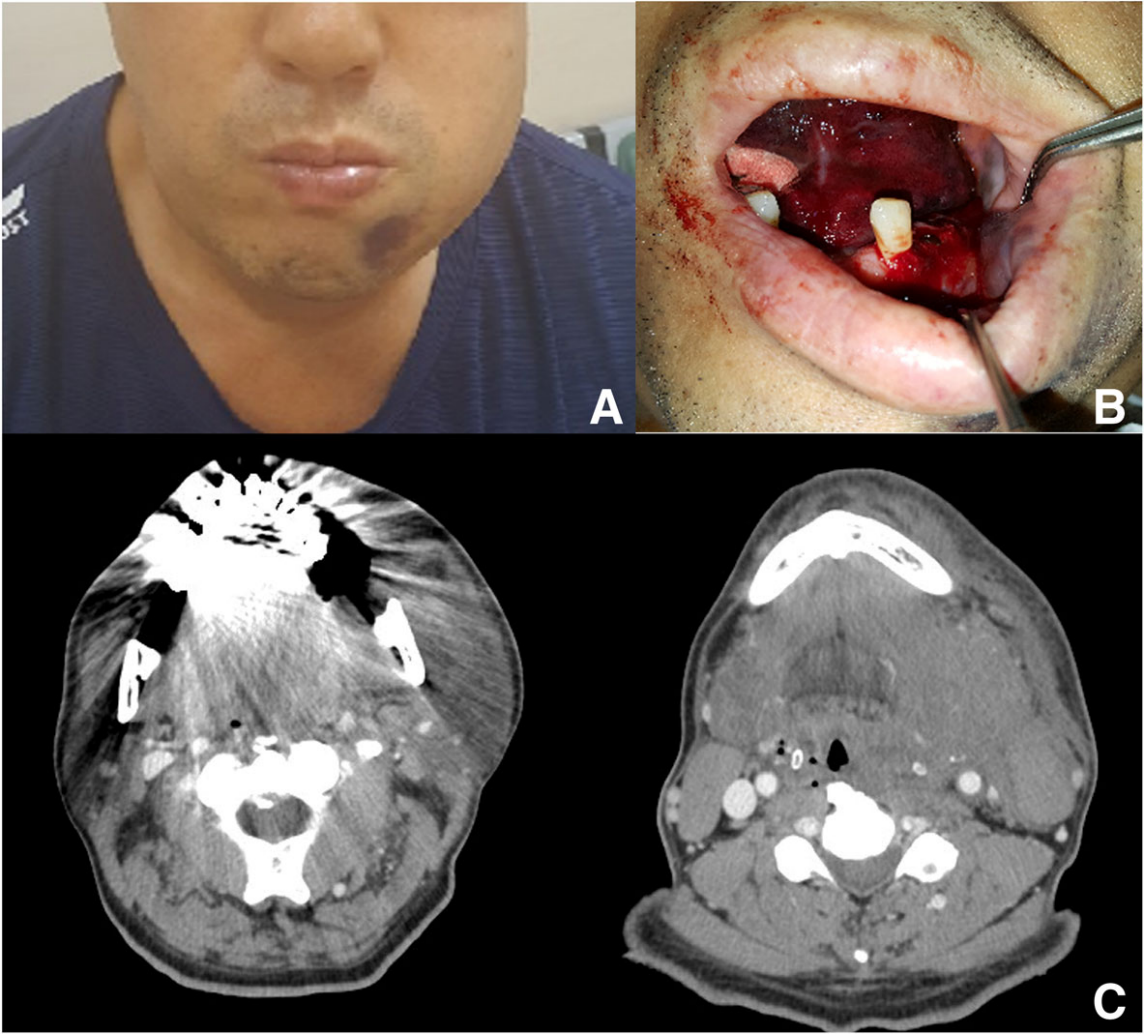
a Facial swelling of an emergency room arrival. b Intra-oral view: mouth floor elevation and bleeding at the implantation site. c CT axial view showed severe narrowing pharyngeal airway due to soft tissue swelling in the left masticatory, mandibular spaces, hypopharynx
